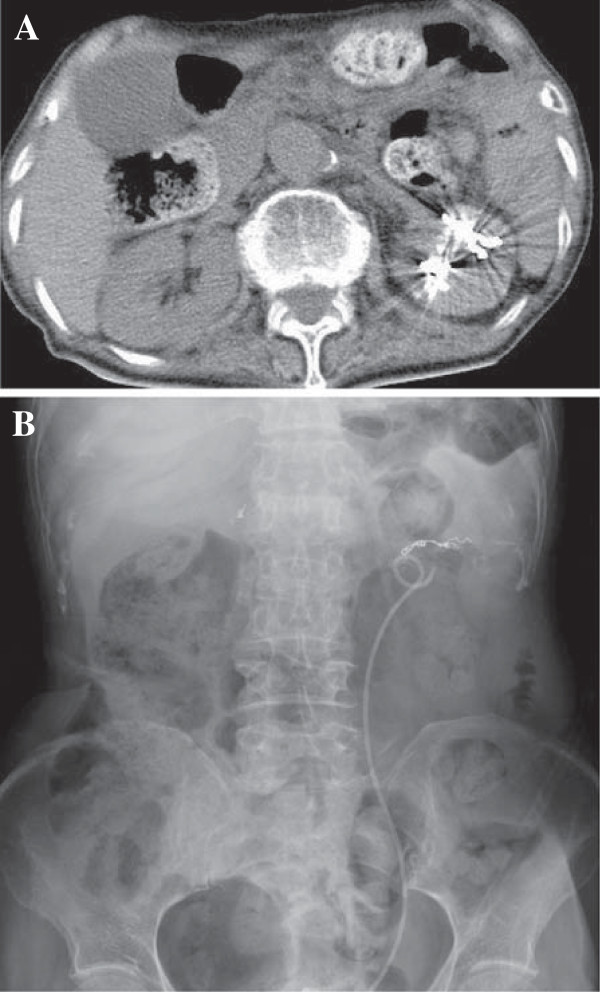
Plain abdominal computed tomography revealed resolution of hematoma in the left renal pelvis and presence of metallic coils (A). Abdominal radiography revealed a well-positioned double-J stent (B).
